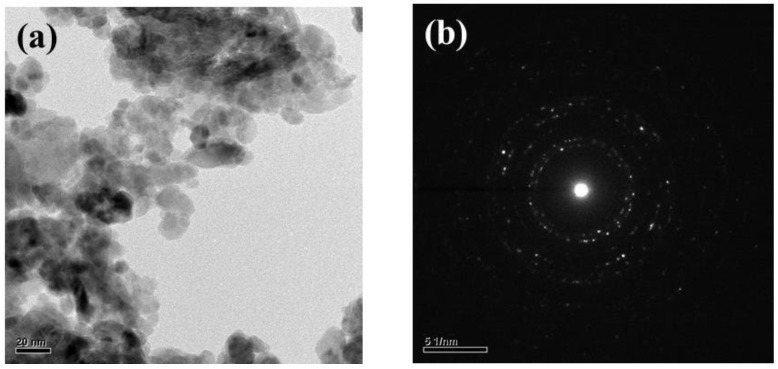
(a) The TEM image of the ZnO powder after ultrasonic grinding and (b) the selected area electron diffraction (SAED) image of the ZnO-NPs.
